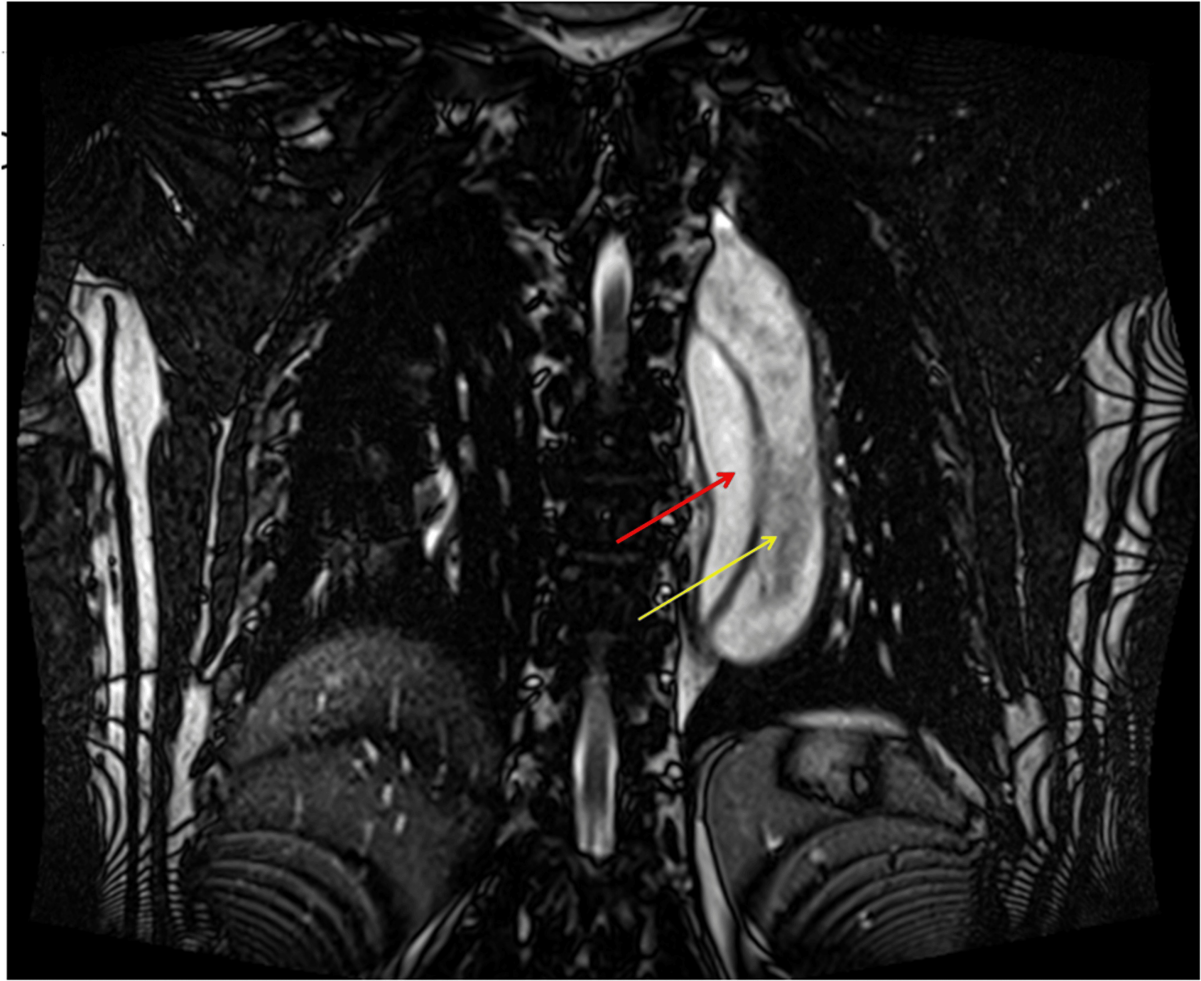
T2-weighted MRI coronal view that demonstrates a dissection of thoracic aorta (approximately 4 cm in diameter) with a smaller true lumen (red arrow) measuring 1.5cm positioned medially, while the larger false lumen (yellow arrow) measuring 2.5 cm positioned laterally
